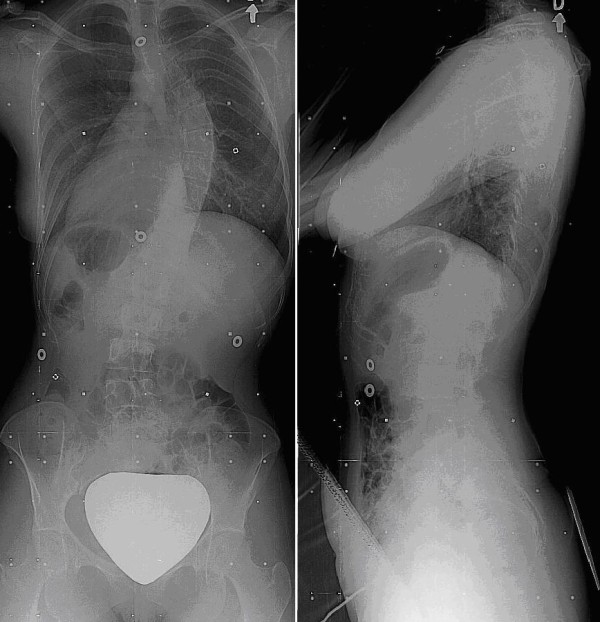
Preoperative posteroanterior and lateral radiographs of the patient.
